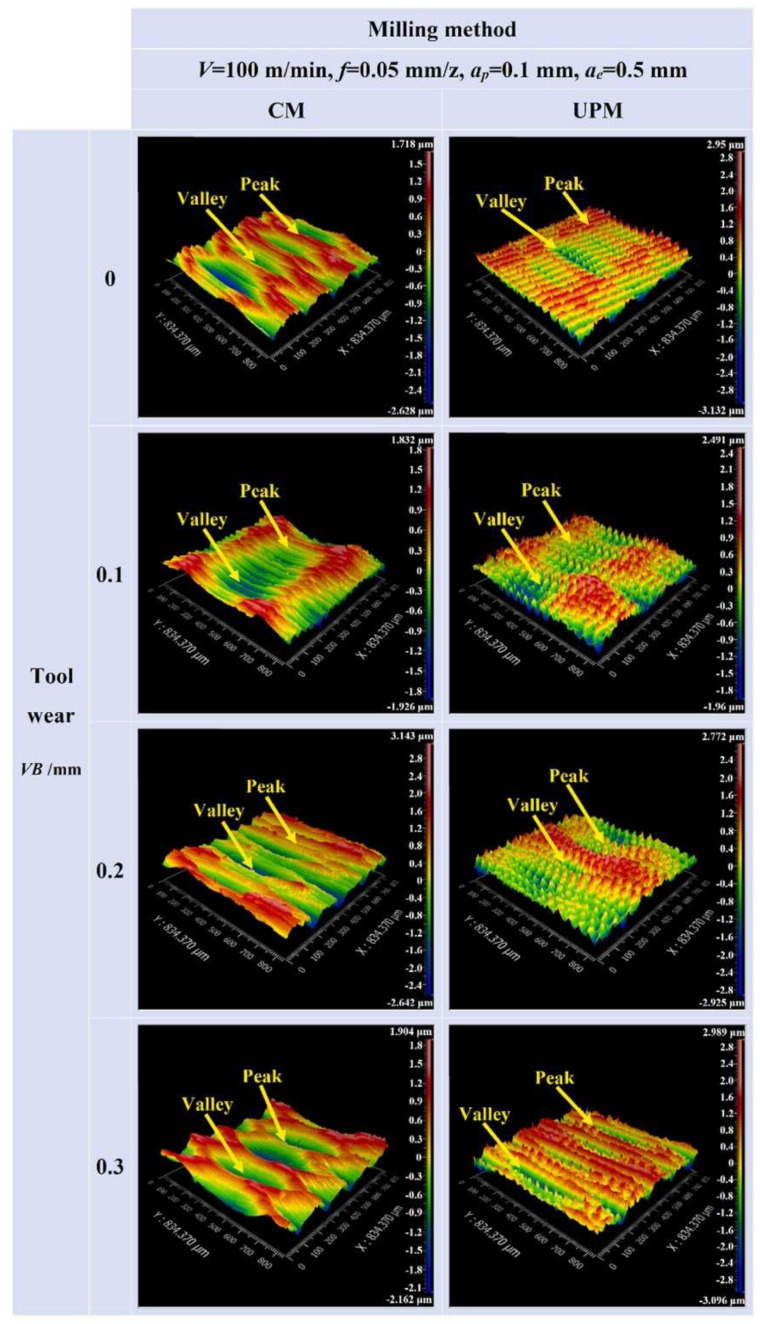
Comparison of surface topographies achieved through conventional manufacturing (CM) and ultrasonic peening milling (UPM) [119].
